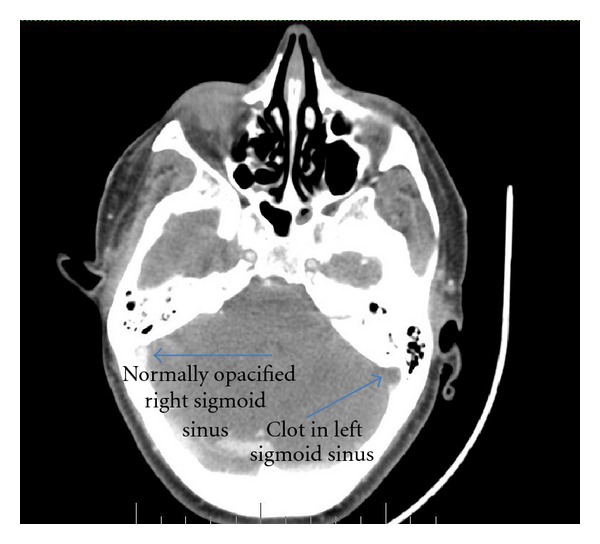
CT scan of the head and neck with contrast demonstrated a thrombus in the left sigmoid sinus.
